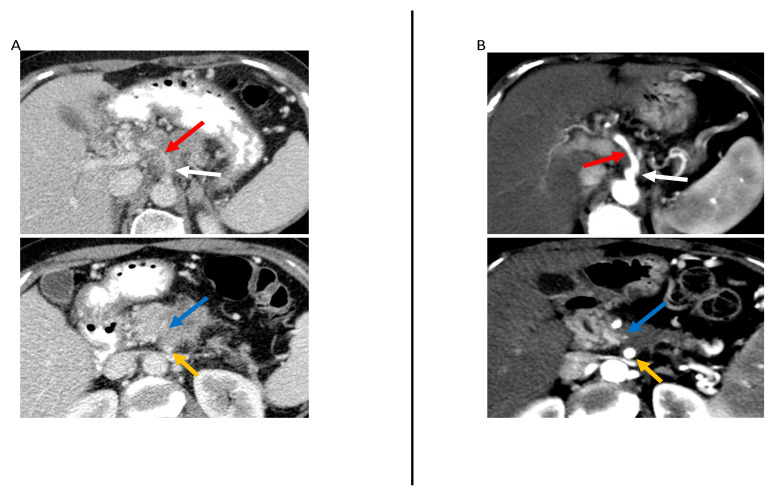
59-year-old woman with locally advanced pancreatic cancer before and after neoadjuvant therapy. Contrast-enhanced CT in the axial plane at primary staging (A) and restaging after neoadjuvant therapy (B) demonstrate an ill-defined pancreatic neck and body tumor (with markedly decreased size after neoadjuvant therapy (blue arrow). At primary staging, the tumor was abutting the celiac artery (white arrow) superior mesenteric artery (SMA) (yellow arrow), causing vessel irregularity and narrowing (red arrow). After neoadjuvant therapy, the SMA abutment by the tumor was overall unchanged, although the artery irregularity and narrowing resolved. Additionally, the soft tissue surrounding the SMA had an enhancement < 30 HU. The patient underwent surgical resection after neoadjuvant therapy and there was no evidence of arterial involvement.
